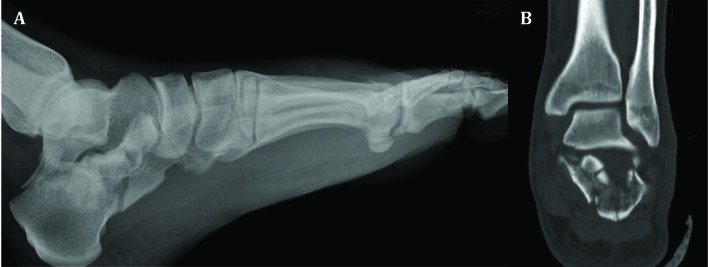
(A) Lateral radiograph and (B) coronal CT section of left ankle showing comminuted joint-depressed fracture of the calcaneus (Sanders type IV).
